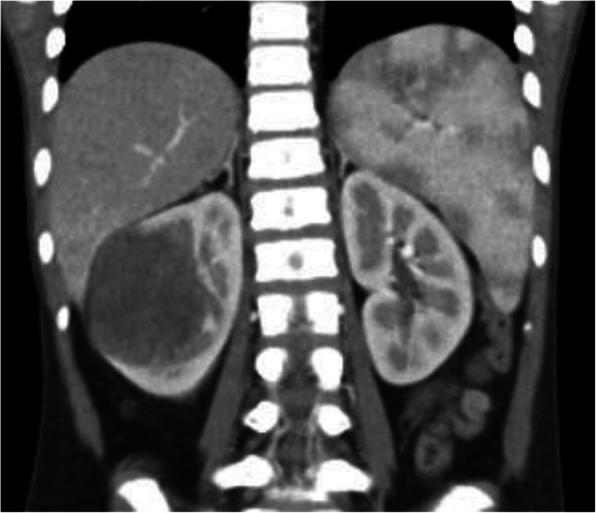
CT abdomen showing right small Wilms’ tumor. The case underwent upfront nephrectomy after surgical consultation
